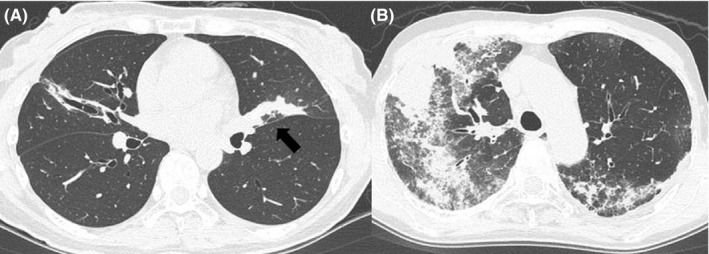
Chest computed tomography in patients with allergic bronchopulmonary mycosis. Chest computed tomography showed shadows filling the bronchial lumen (A, arrow) and pulmonary consolidations (B)
